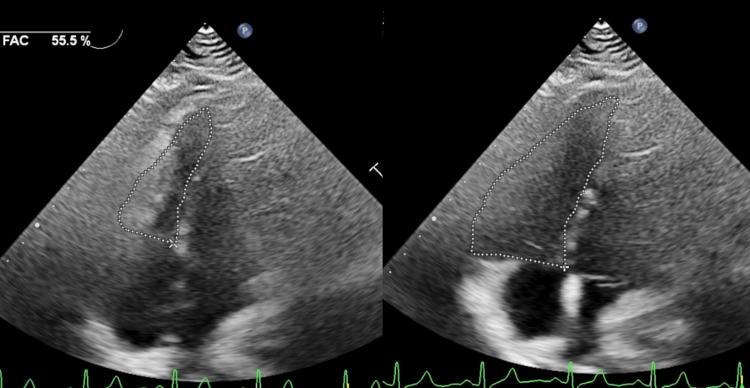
Echocardiography of right ventricular function five years after re-reoperation FAC: fractional area change
